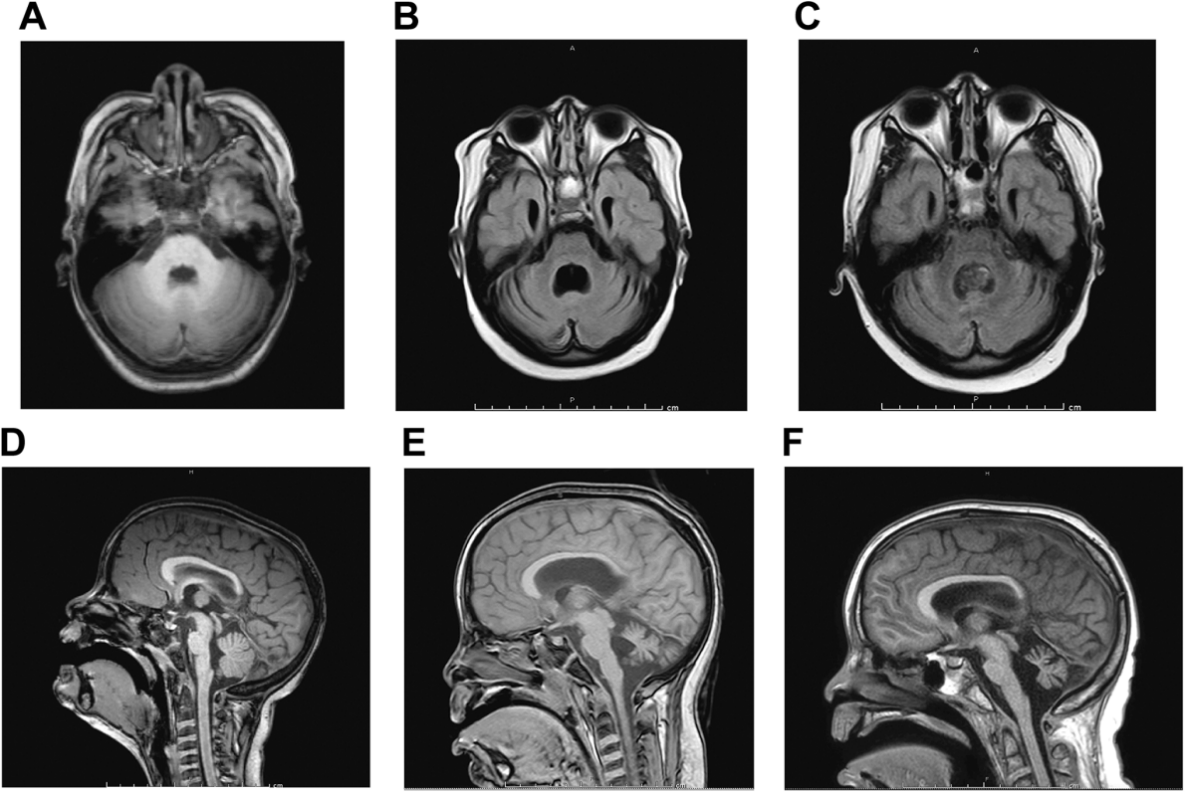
MRI axial and sagittal FLAIR imaging. A, Pre-PCD axial T1 image at time of ataxia
